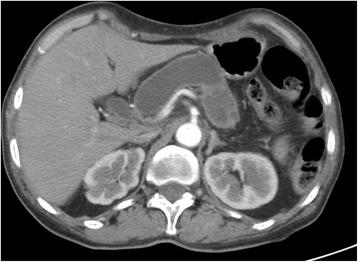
Abdominal CT scan showing the dilation of the pancreatic main duct
